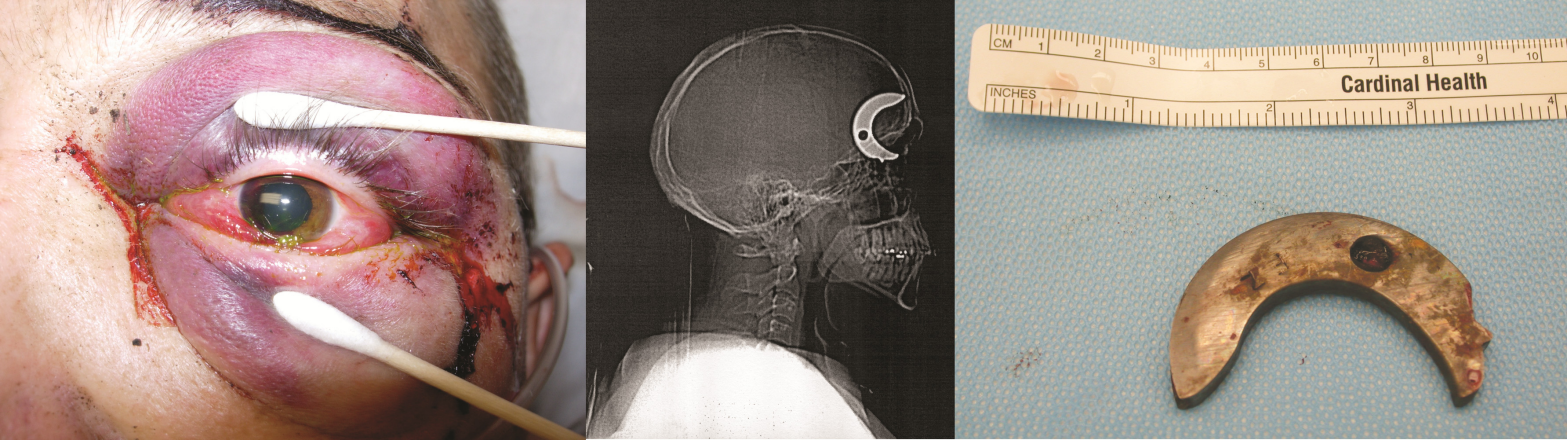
a *Left*: External photograph of left eye and periorbital area. Note the laceration lateral and inferior to the lateral canthus. b *Center*: Sagittal plain film radiograph of the head and cervical spine shows a large sickle-shaped metallic density extending through the left orbital roof into the left frontal lobe. c: *Right*: Metallic object shown in b immediately following surgical removal.
